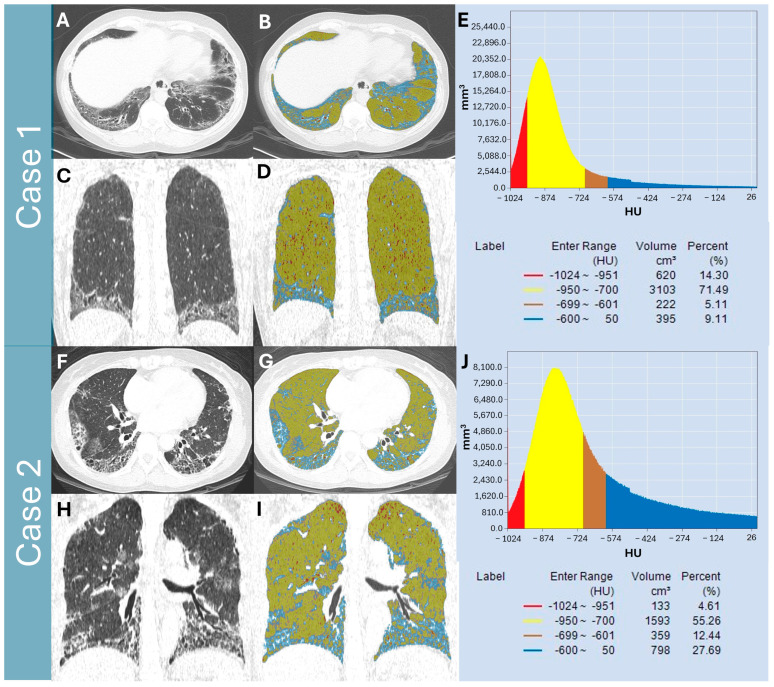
Two representative cases of quantitative lung parenchymal radiodensity measurement. Representative focal histograms demonstrating quantitative CT analysis using predetermined Hounsfield unit (HU) thresholds. Normal lung volume (NLV%, yellow) was defined as regions with densities between −950 HU and −700 HU. Fibrotic lung volume (FLV%) was calculated as the density ranges from −600 HU to +50 HU (blue). Case 1: nonspecific interstitial pneumonia in a 46-year-old female diagnosed with primary Sjogren syndrome. The high-resolution CT axial image (A) and coronal image (B) reveal a mixture of ground glass opacity (GGO) and reticulation. The color-overlayed CT axial image (C) and coronal image (D) provide visual assessment of lung lesions. The histogram (E) showed the quantitative results for each CT-derived index. Case 2: nonspecific interstitial pneumonia in a 50-year-old female diagnosed with dermatomyositis. The CT axial image (F) and coronal image (G) reveal GGO, fine mixed coarse reticulations, and focal fibrotic consolidation. The color-overlay axial image (H) and coronal image (I) demonstrated the corresponding lesions. The histogram (J) showed the quantitative results for each CT-derived index.
